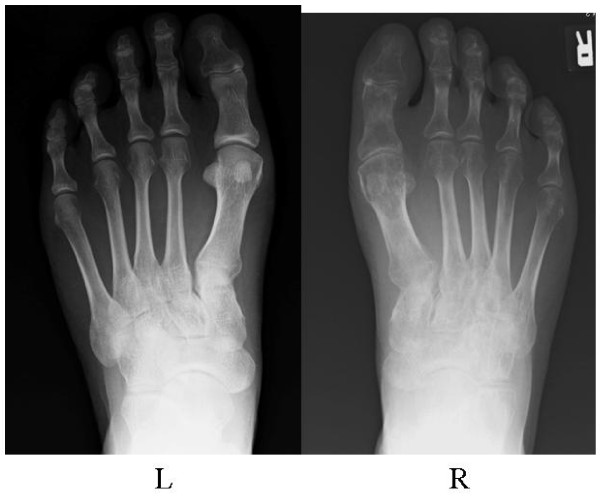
Radiographs of the both feet after treatment. The regional osteoporotic changes in the right foot (Figure 5A) were markedly improved at 32 months after the start of bisphosphonate treatment.
